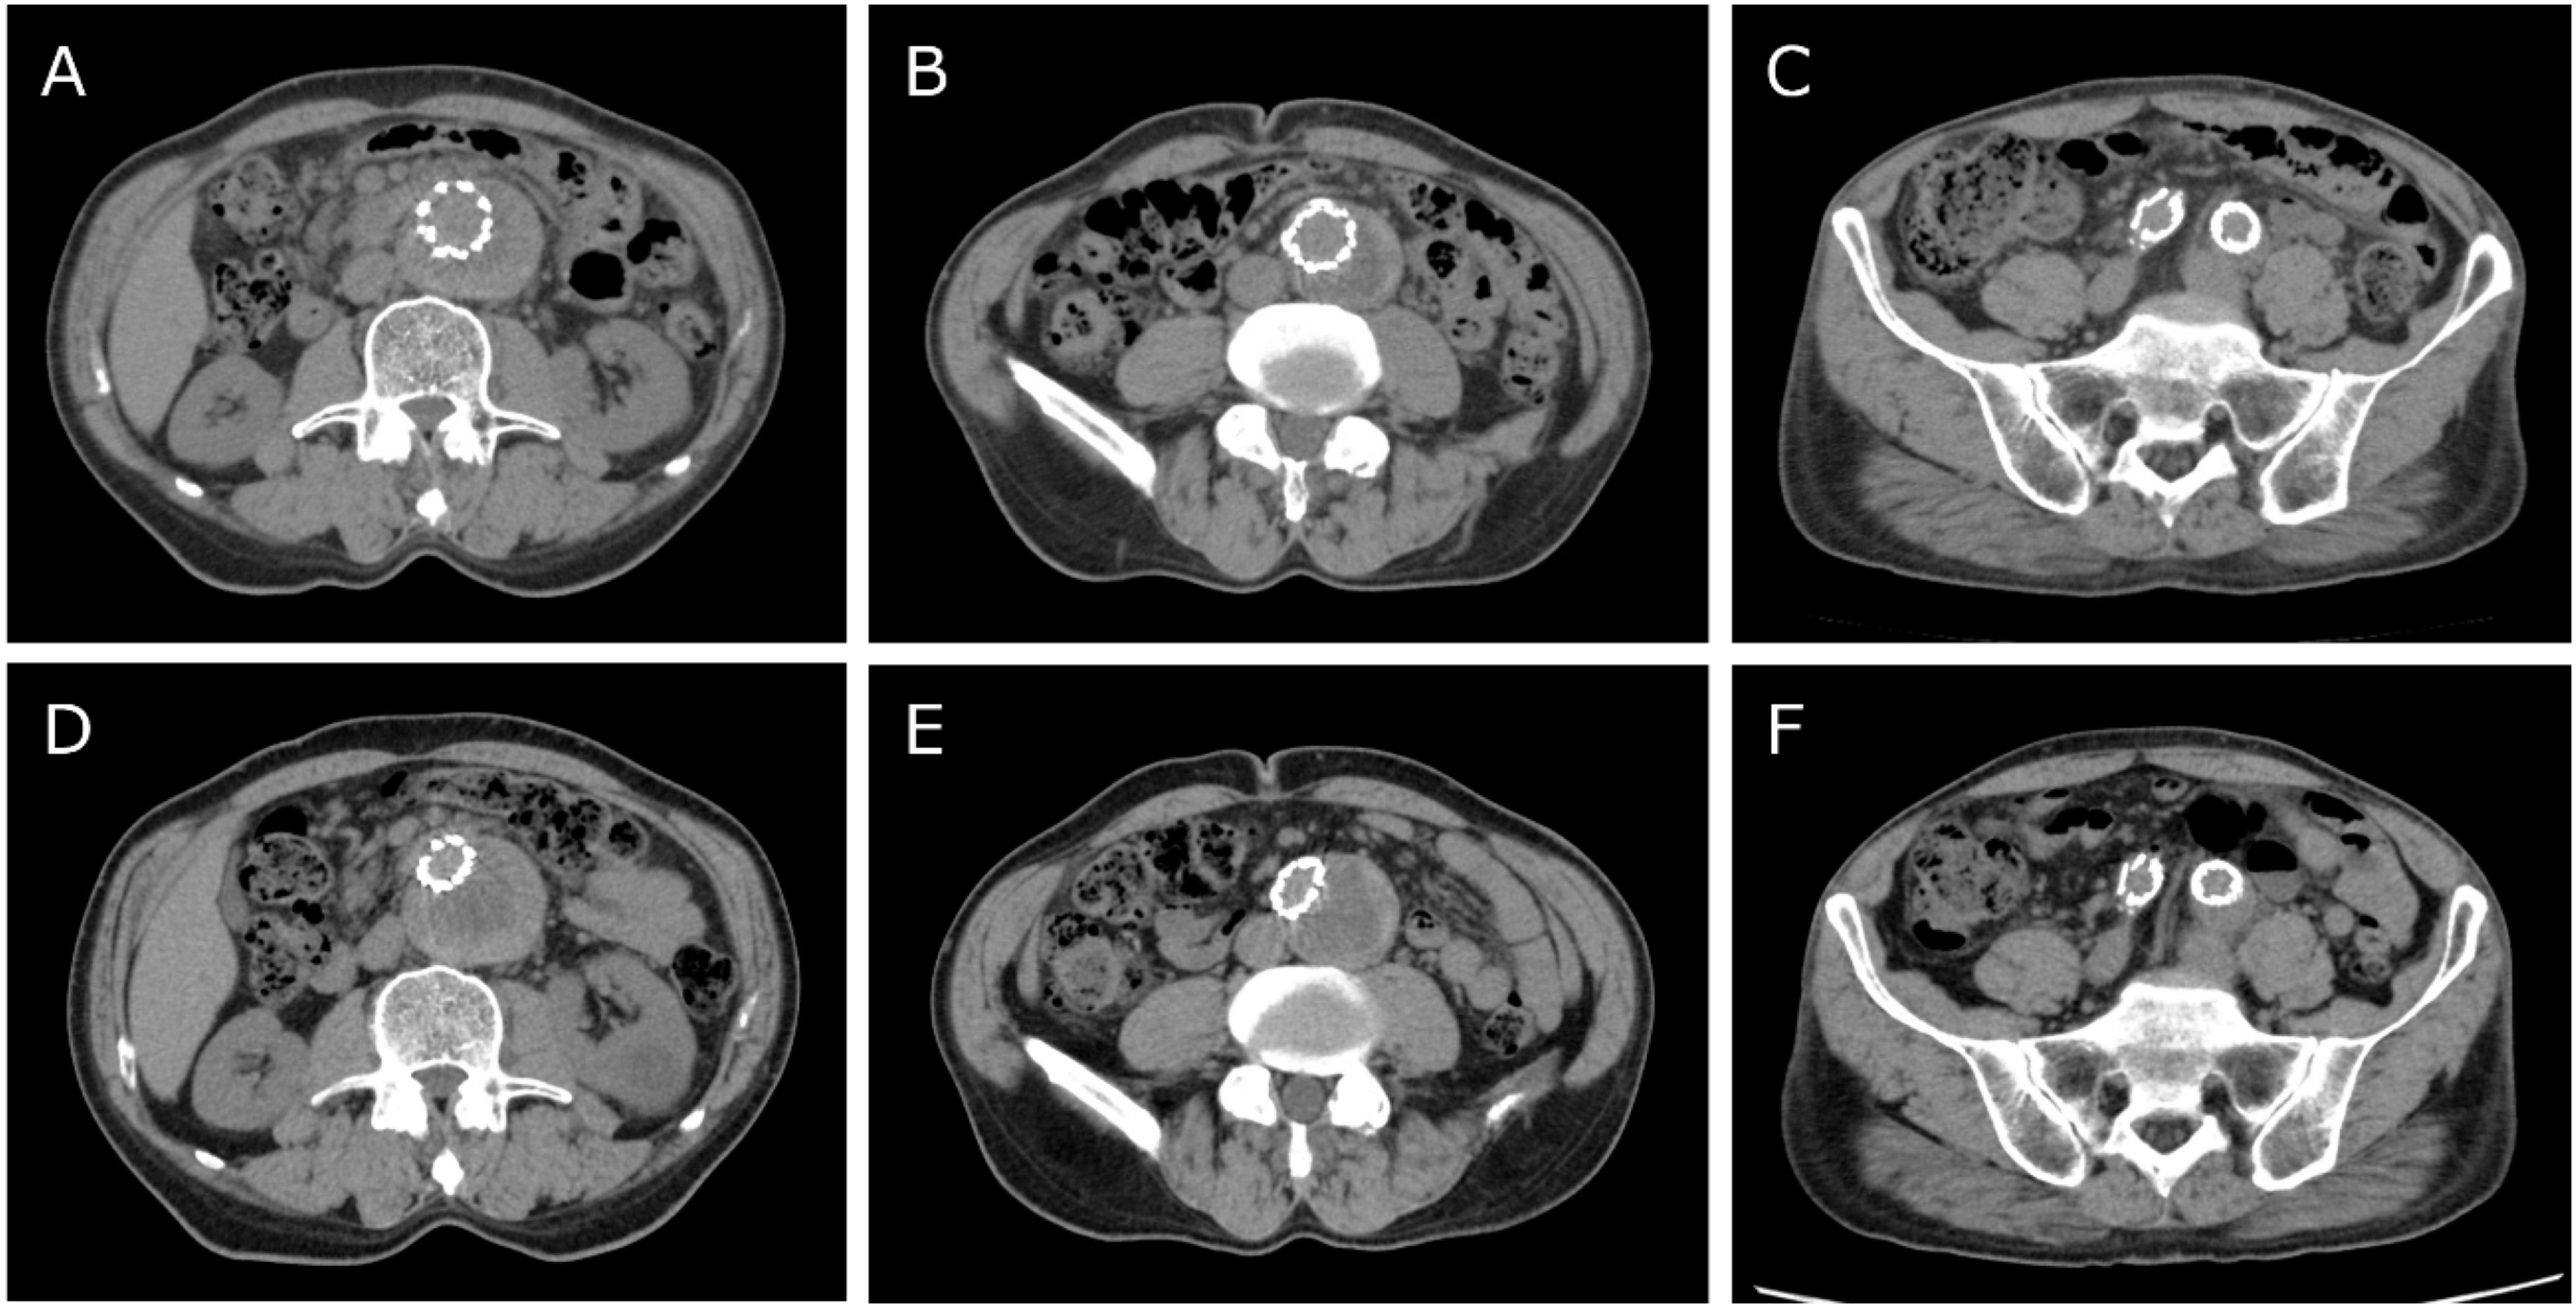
Fig. 4 Plain computed tomography (CT) 7 months after the procedure showing a slight reduction in the diameter of the abdominal aorta and good expansion of the stent graft (A, B, C) compared with the same level of CT 1 month after the procedure (D, E, F).
